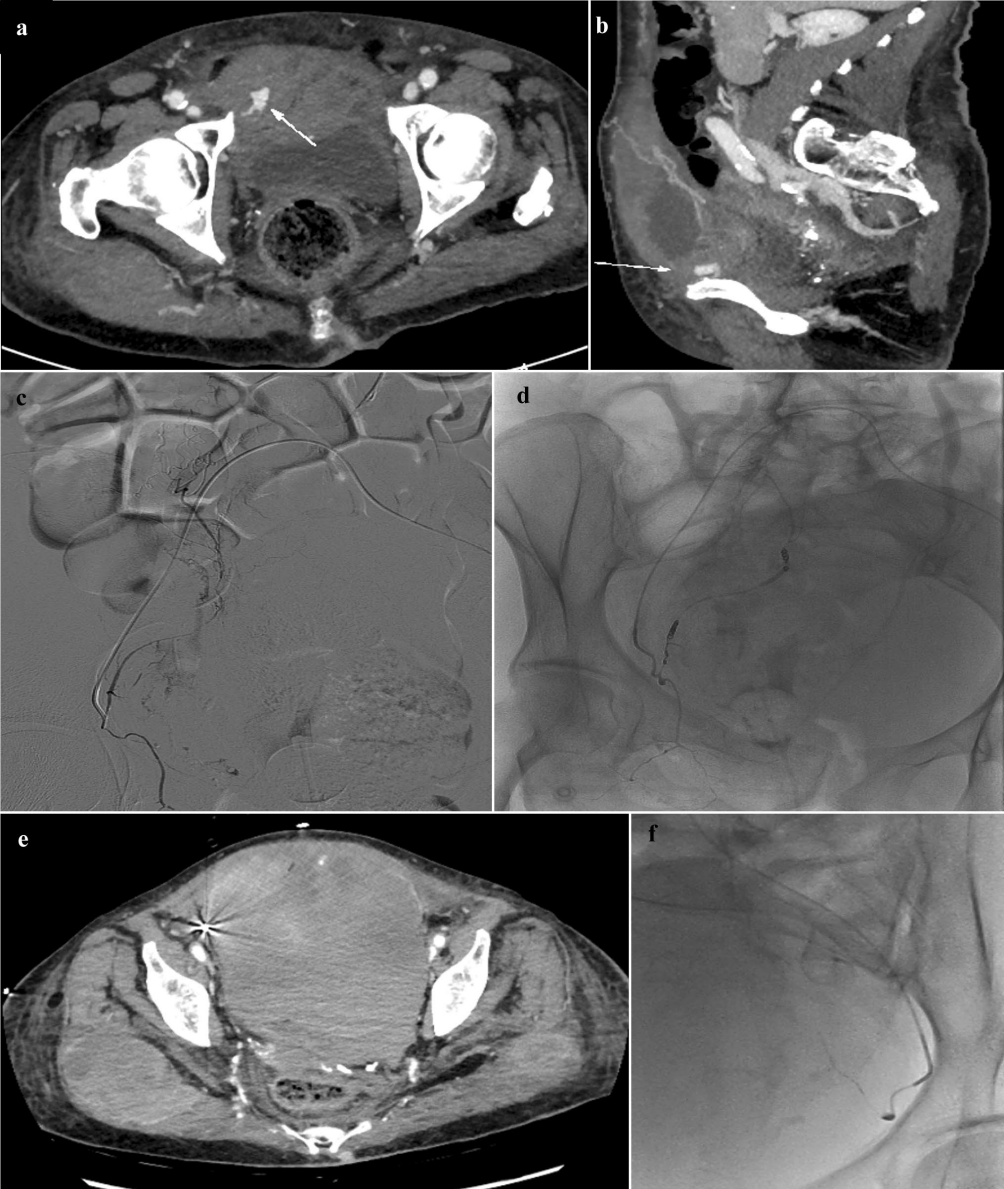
CT and DSA images of a case of recurrent spontaneous AWH in a SARS-CoV-19 patient. Axial (a) and sagittal (b) CT images after contrast-enhanced acquisition at arterial phase showed a large hematoma within the right rectus abdominis with active arterial bleeding. At DSA, multiple contrast extravasations from right inferior epigastric artery were detected (c). PTE procedure was performed with “front and back” technique using 2 and 3-mm microcoils (d). Twenty-four hours after PTE, a recurrent AWH with pelvic spreading was detected at contrast-enhanced CT (e). At DSA, the left inferior epigastric artery showed active extravasation and embolized using 2-mm microcoils (f)
